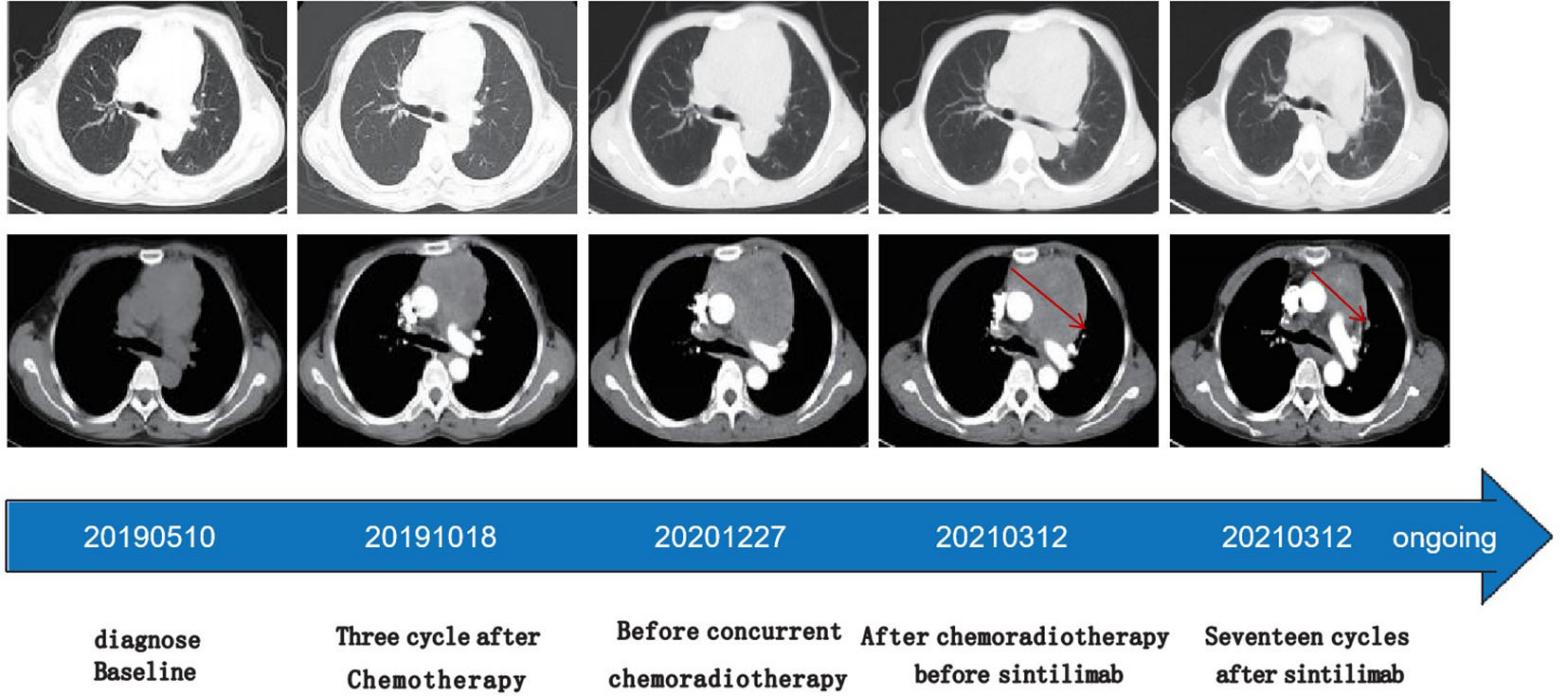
Time line of treatments of the patient and changes in CT scan of thymic carcinoma during treatments Chest computed tomography (CT) revealed a clearly reduced the size of anterior mediastinum lesion indic- ated by red arrows, with a maximum diameter decreasing from 99 mm to 52 mm.
